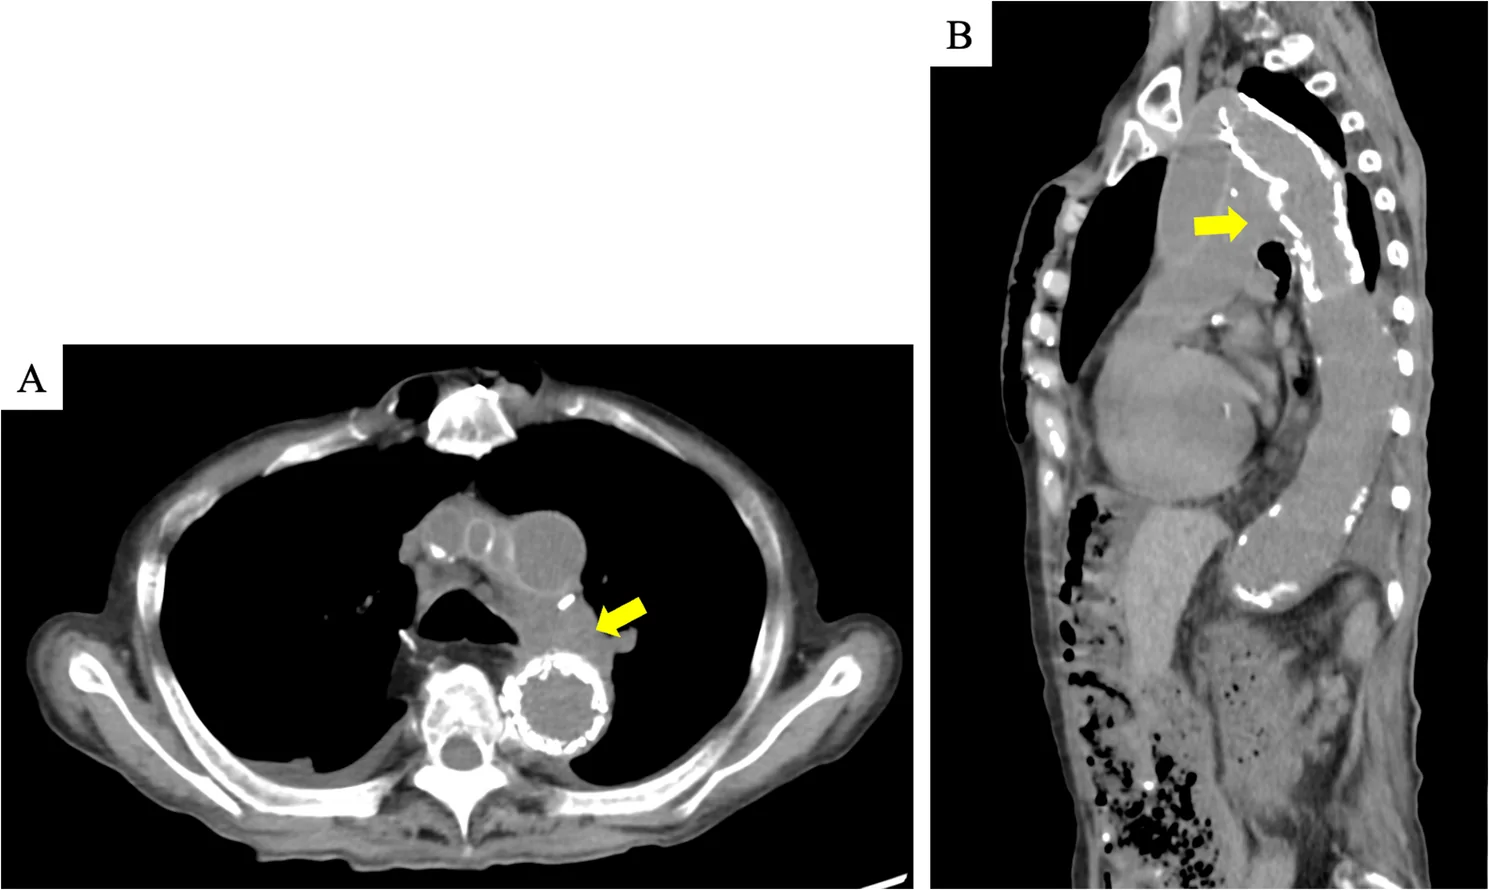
Postoperative follow-up non-contrast CT obtained after staged reconstruction. A Axial non-contrast CT image obtained after staged treatment demonstrating resolution of the previously observed peri-aortic gas and fluid collection seen in Figure 2, with no residual ectopic gas around the thoracic aorta, suggesting improvement of perigraft inflammatory changes. B Sagittal non-contrast CT image showing the thoracic aortic stent graft in situ after TEVAR and presternal oesophageal reconstruction, with no peri-graft gas or radiological evidence of persistent mediastinal infection
